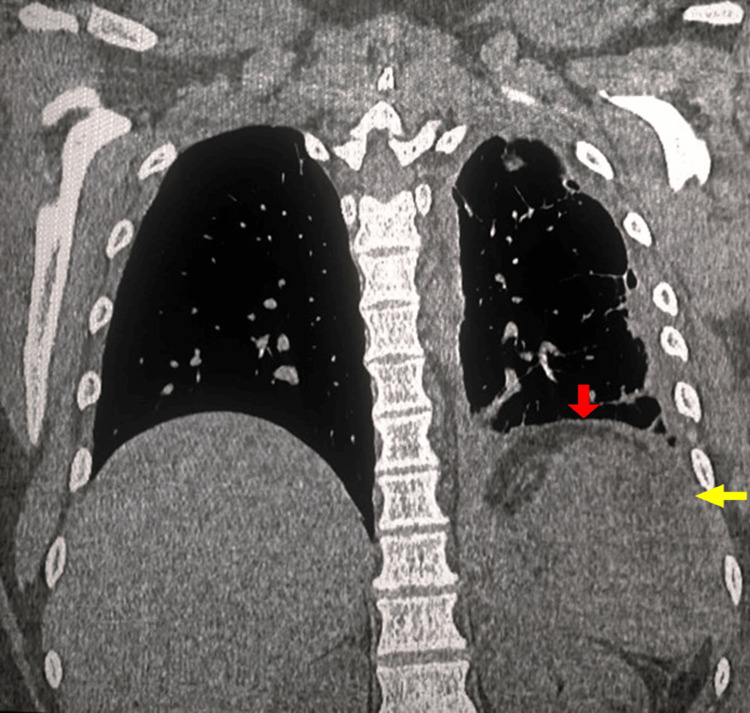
CT taken two weeks postoperatively demonstrated that the splenic hematoma (yellow arrow) had decreased in size and the pleural effusion had resolved (red arrow).
